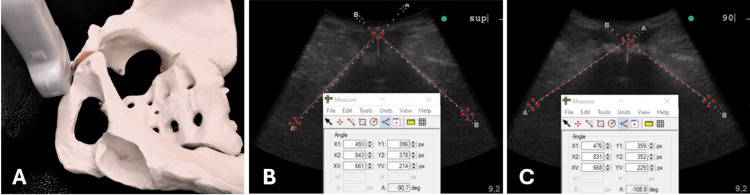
Perineal probe position and ultrasound PAA measurement. The optimal position of the probe on the perineum to visualize the ischio-pubic branches of the pubic arch angle is on the lower edge of the pubic symphysis at 45° tilt. The ultrasound PAA measurements of a multiparous non-pregnant supine patient demonstrate an amplitude difference of 18.2° between the hip extended and 90° hip flexed positions. A: The correct probe position and orientation on the perineum. B: Ultrasound image in the supine position (sup), PAA= 90.7°. C: Ultrasound image in the 90° flexed leg position (90), PAA= 108.9°. PAA: Pubic arch angle Image credit: Marco Siccardi.
